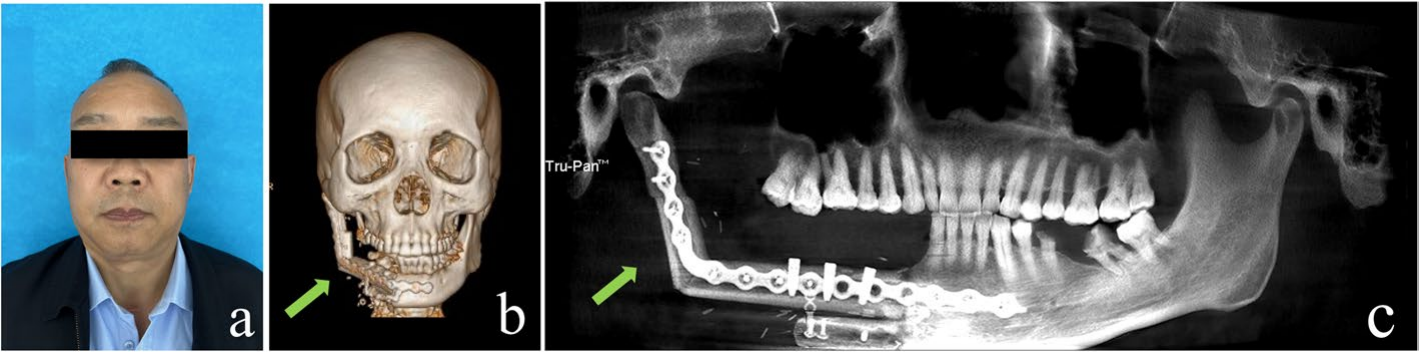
Post-operative follow-up image of a 54-year-old male with SCC of the right mandible gingiva: a Post-operative photo; b Post-operative CT; c post-operative CBCT. The arrow points to the reconstructed mandibular portion of the fibula flap
